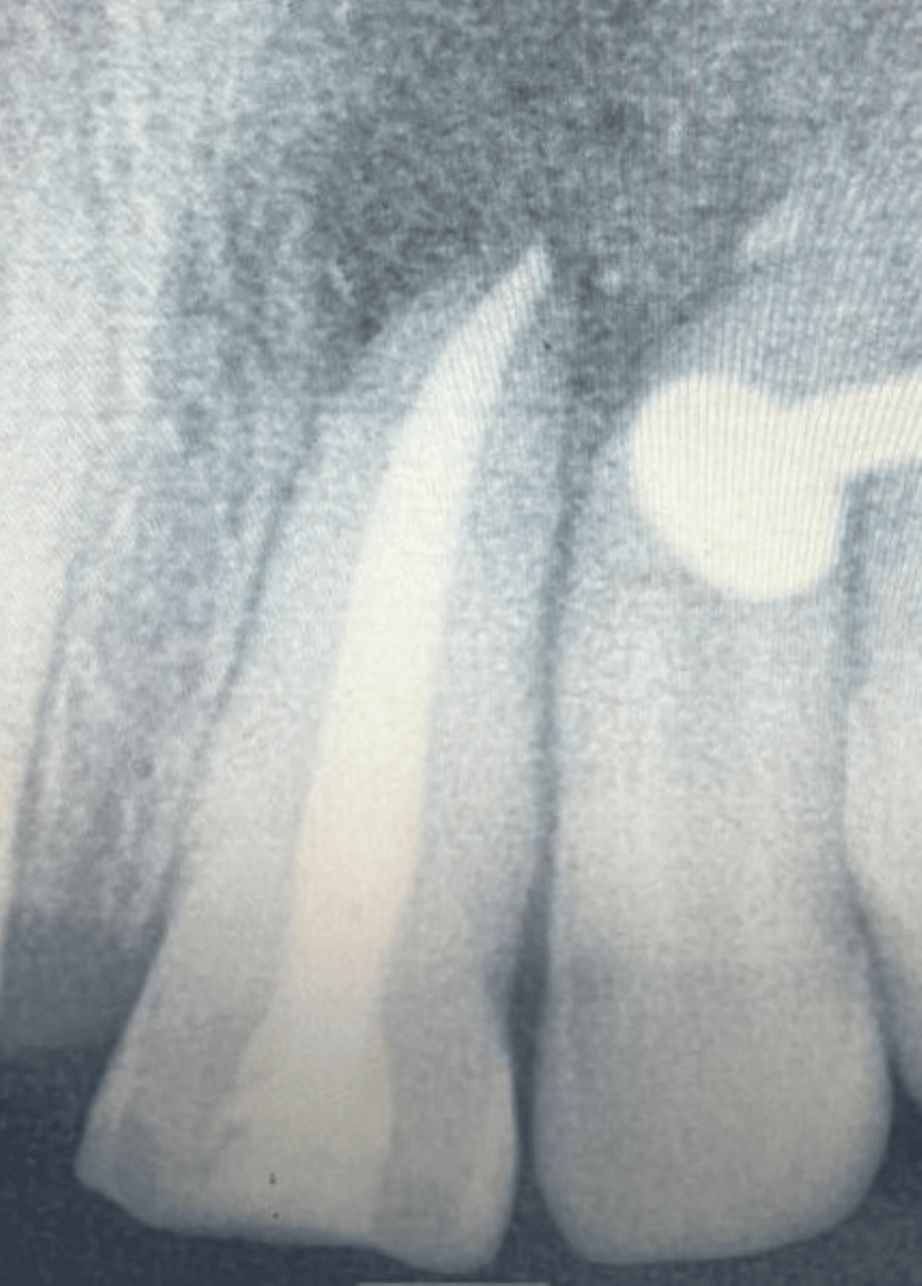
Radiograph taken on during the follow-up session at three weeks; a nosepin visible as an artifact.
